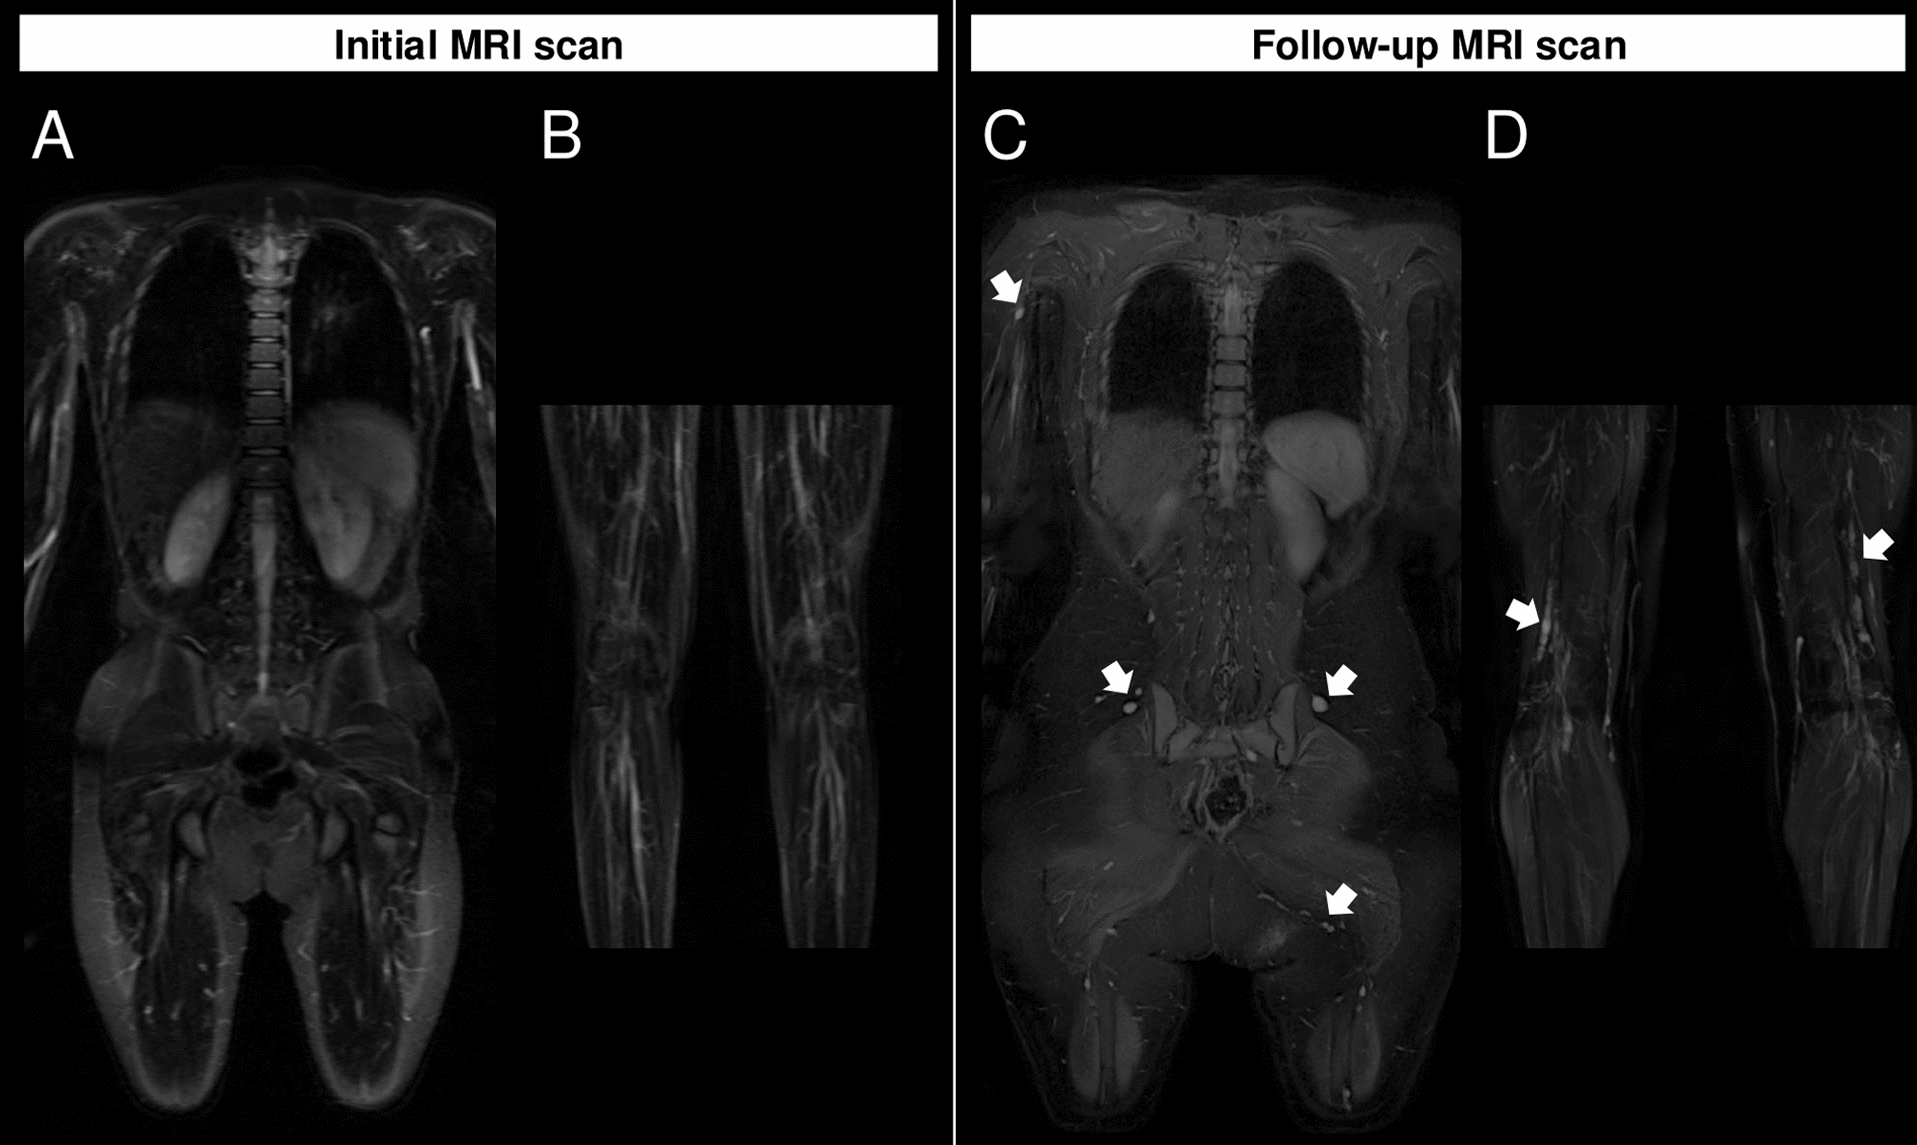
Patient example 2 with newly detected PN during follow-up MRI. A, B Coronal T2-weighted, fat-saturated MRI sequences of the body and the upper limbs acquired at 1.5T in a 9-year-old female, who displayed no peripheral nerve sheath tumors at the initial scan in 2007. C, D Follow-up examination in 2020 at the age of 22 shows multiple newly developed smaller neurofibromas along all larger peripheral nerves (arrows) on the coronal T2-weighted, fat-saturated MRI sequence of the body and the upper limbs at 3T
